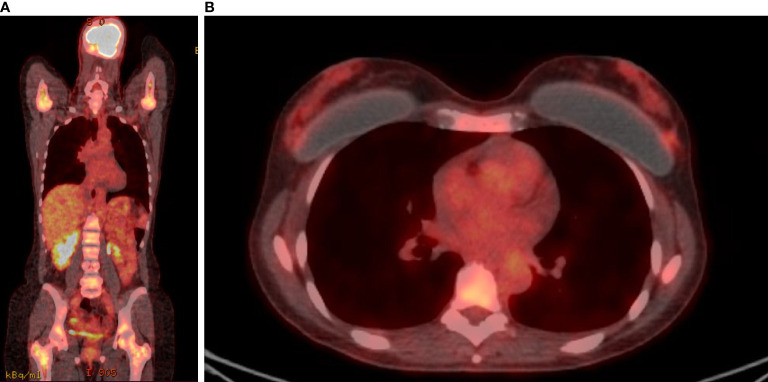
FDG-PET/CT. FDG uptake was detected in almost all bone segments (A) and in breast lesions (B) before treatment.
